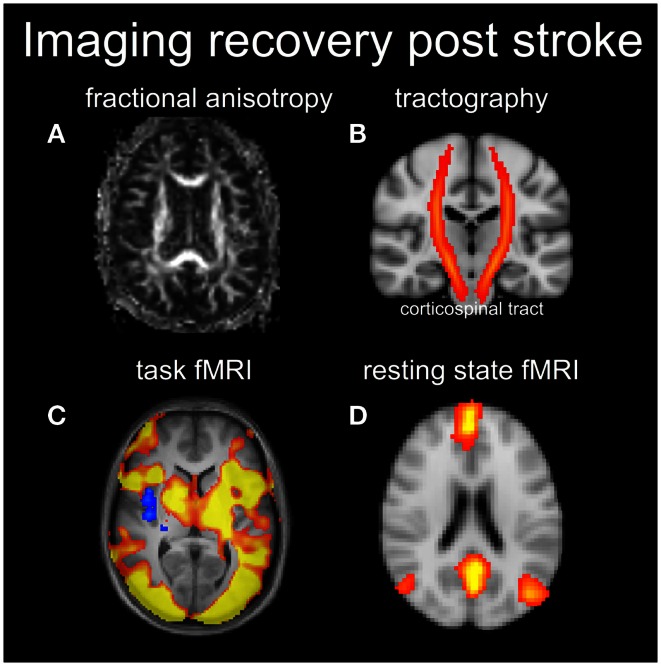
Diffusion tensor imaging can be used to visualize the fractional anisotropy (FA) (A) in white matter (brighter signal means higher FA) or produce the white matter tracts (B). In this case an atlas based corticospinal tract is shown in red and overlaid on a coronal anatomical image. Functional MRI results shows task-based activation (C) and a resting-state network corresponding to the default mode network (D).
